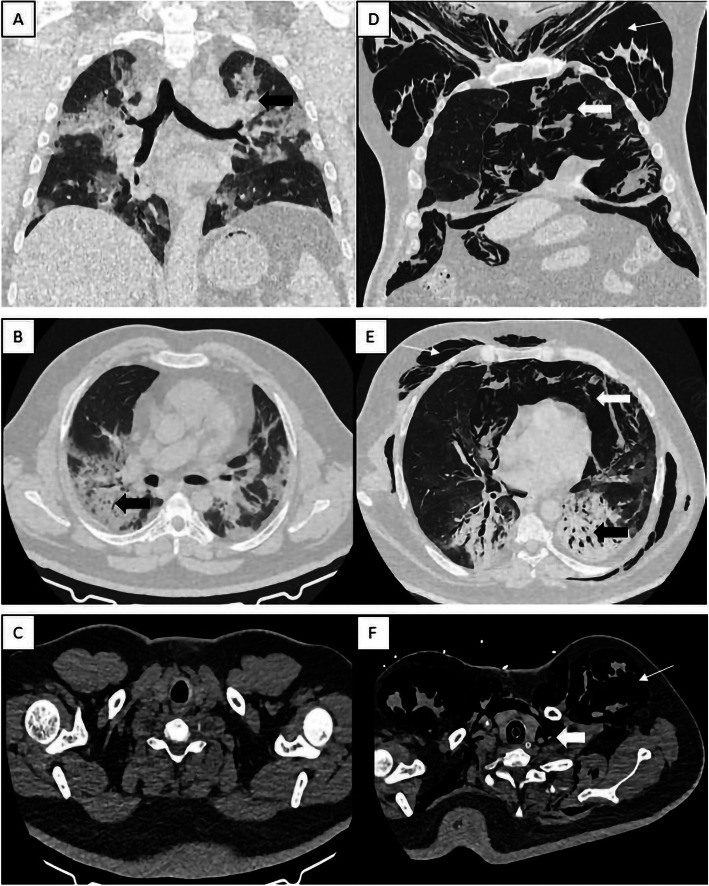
Pre (a, b, c) and post (d, e, f) intubation HRCT chest images demonstrating the presence of consolidation (black block arrow) in bilateral lung fields. Presence of diffuse subcutaneous emphysema (white arrow) is noted along bilateral chest wall extending into arms and back along with pneumomediastinum (white block arrow) extending up to the paratracheal area
